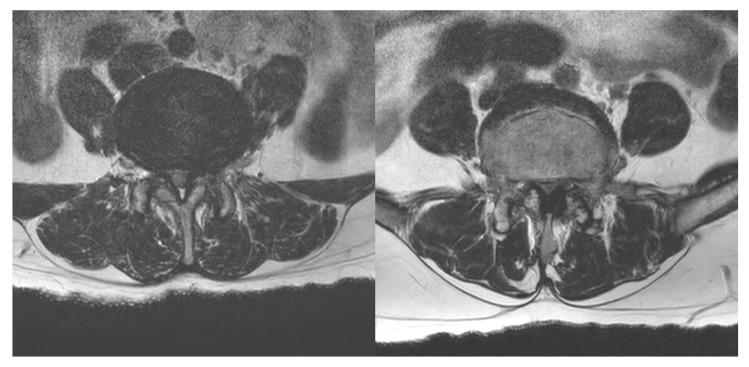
Severe (left) and extreme (right) lumbar canal spinal stenosis on T2-axial magnetic resonance imaging.
